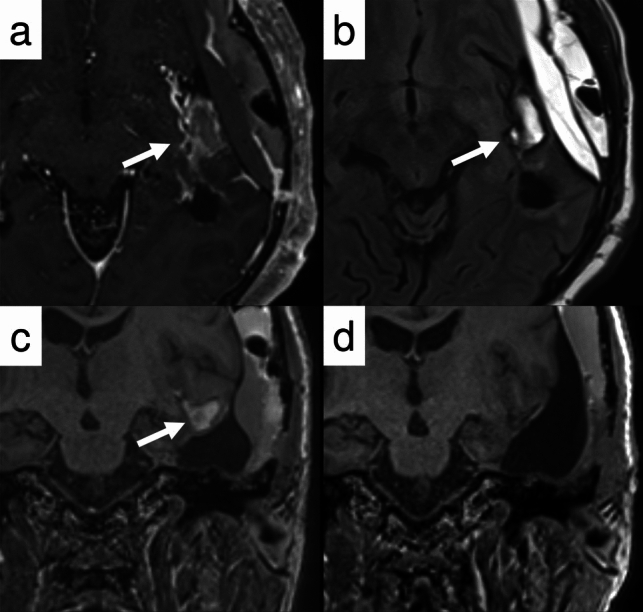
Postoperative imaging of a 72-year-old man with *IDH*-wildtype glioblastoma. a Contrast-enhanced T1WI shows a hyperintense lesion (arrow) adjacent to the resection cavity. b FLAIR sequence shows the same lesion as hyperintense with a smooth intersulcal contour. c Coronal T1WI confirms the lesion is confined between cerebral sulci. d Follow-up post-contrast T1WI shows complete resolution of the lesion, consistent with an intersulcal hematoma
